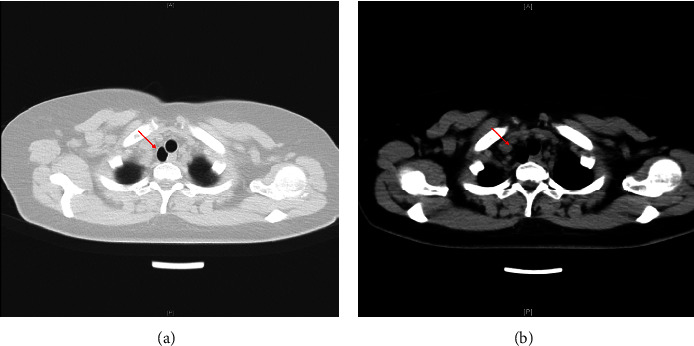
Chest CT cross-sectional images of lung window (a) and mediastinal window (b) (the arrow indicates the TD).
